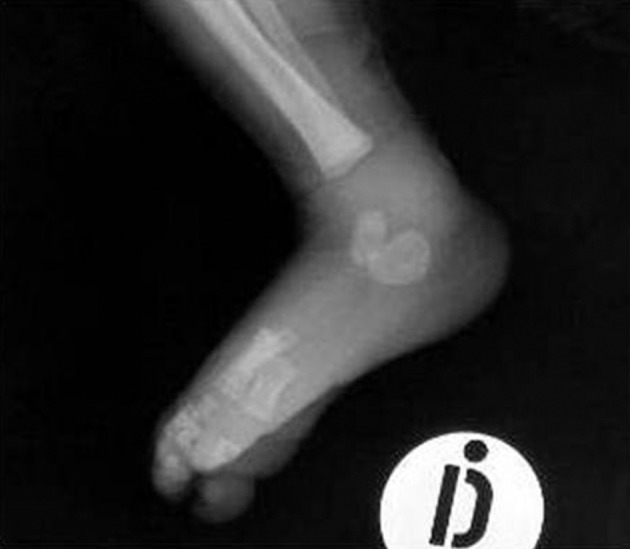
X-ray of the right ankle on admission at 11 days of age
